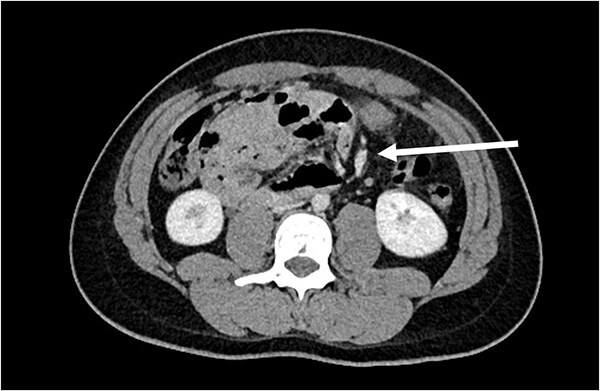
Axial CT image showing the clustered small bowel loops pushing the superior mesenteric vessels toward the left hemiabdomen (arrow points to the superior mesenteric vessels).
